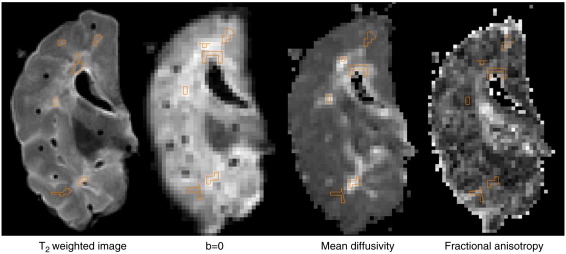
T*_2_-weighted (*T*_2_w) MRI, *b* = 0, mean diffusivity (MD) and fractional anisotropy (FA) maps of *post mortem* multiple sclerosis brain. On *T*_2_w MRI of a coronal brain slice, seven exemplary regions of interest (ROI; marked in orange) were identified as either normal-appearing white matter (NAWM) or white matter lesions (WMLs). ROIs were visually matched with respective ROI on *b* = 0 images, and then co-registered to the MD and FA maps. One of the five WMLs seen on *T*_2_w MRI (asterisk) could not be detected on diffusion maps.
